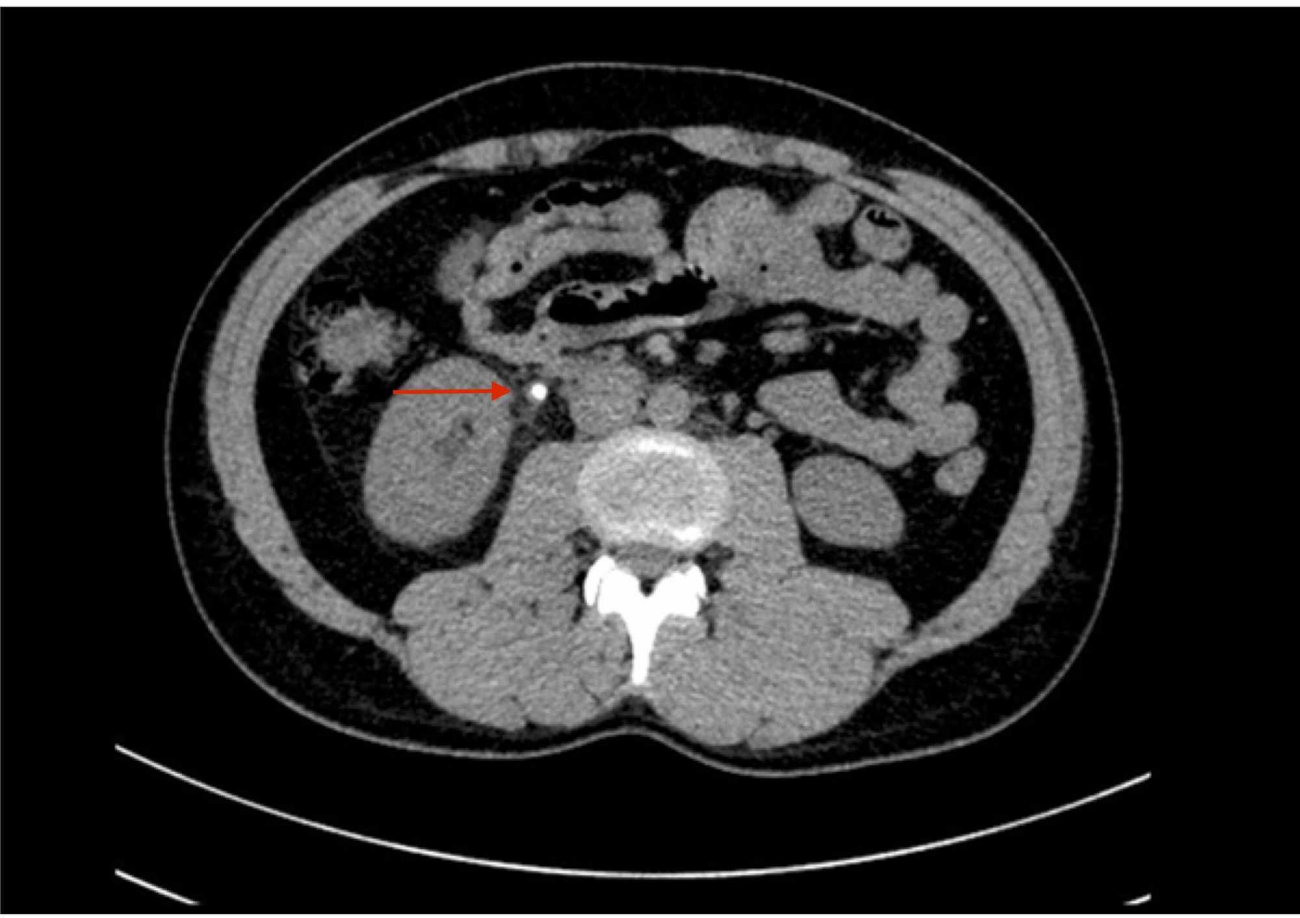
Axial non-contrast DECT image showing a calculus in the right proximal ureter with perinephric and periureteric fat stranding DECT: dual-energy computed tomography
